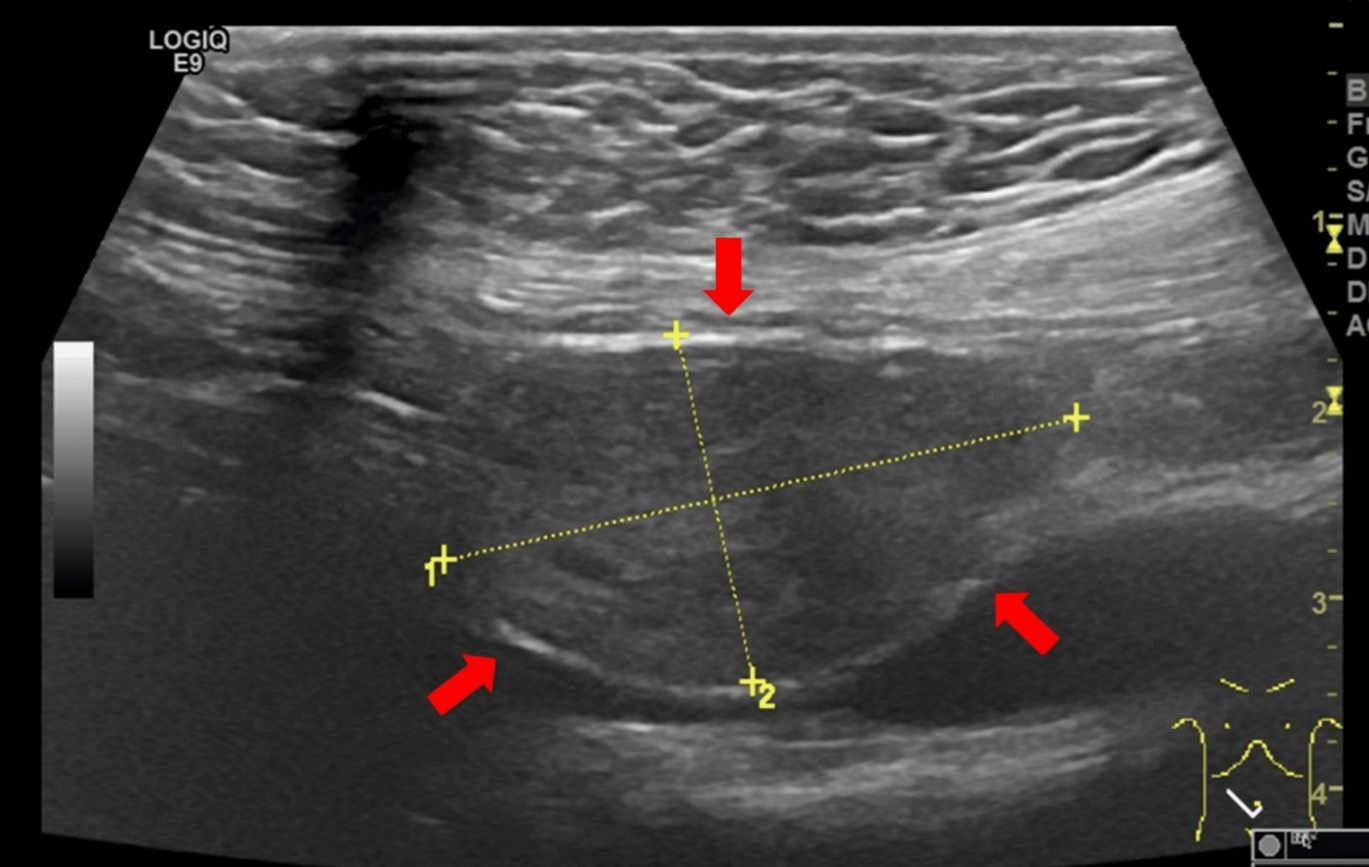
Abdominal ultrasound showing a retroperitoneal mass. Abdominal ultrasound showing a well-circumscribed hypoechoic solid lesion in the interaortocaval region of the retroperitoneum (red arrows), measuring approximately 24 mm in its greatest diameter, as indicated by the caliper measurements.
